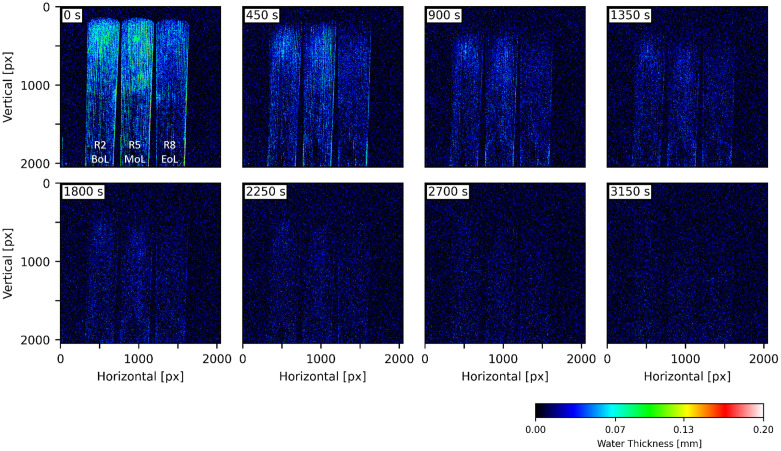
Water thickness images derived from in situ neutron radiography of 2-min-wet reeds drying. Three reeds are R2 (BoL), R5 (MoL), and R8 (EoL) from left to right in each timestamp. The reeds were immersed in water for 2 min before imaging.
